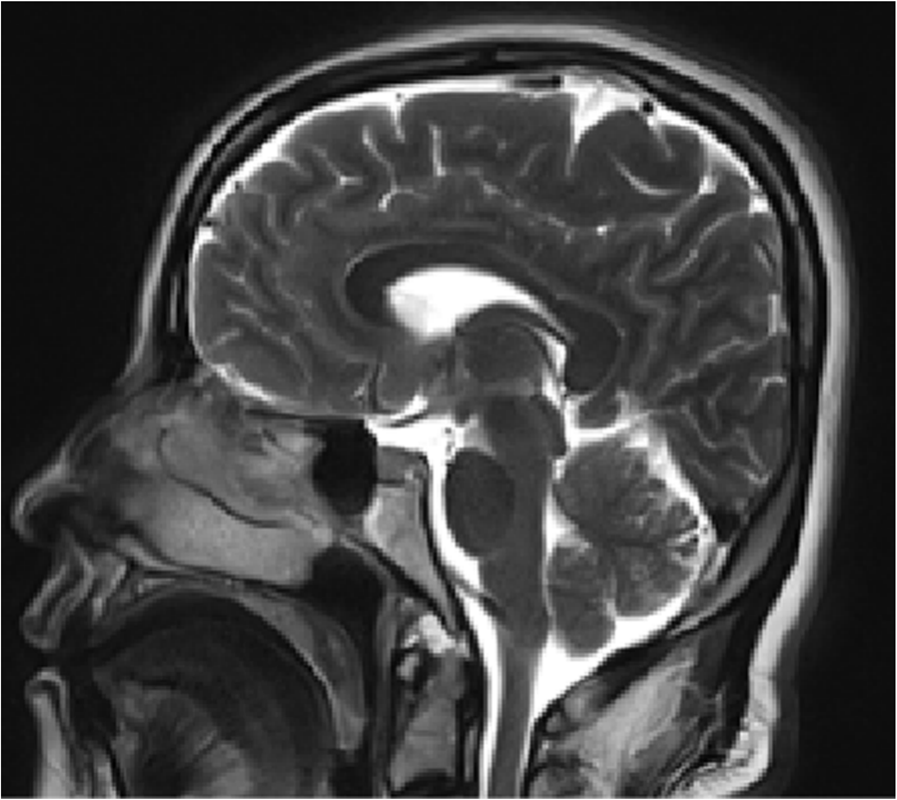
Sagittal T2 magnetic resonance imaging (MRI) of the brain (05/01/2015)
